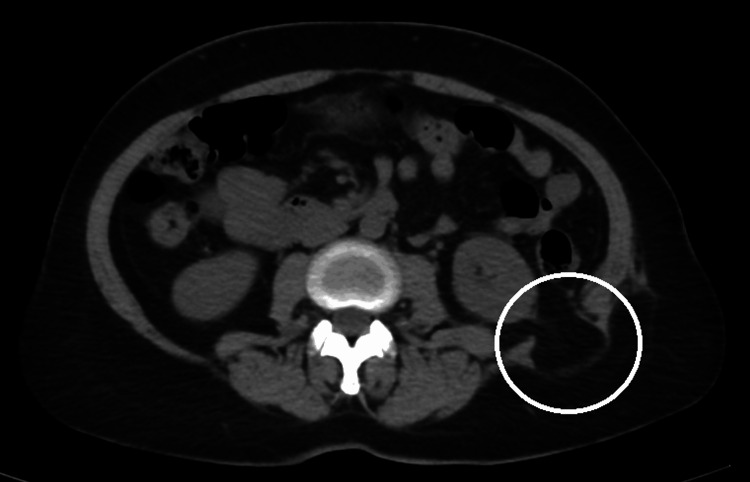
Cross-sectional CT images showing a lumbar hernia defect delineated by the circle, with herniating retroperitoneal fat and associated surrounding musculature. CT: computed tomography.
